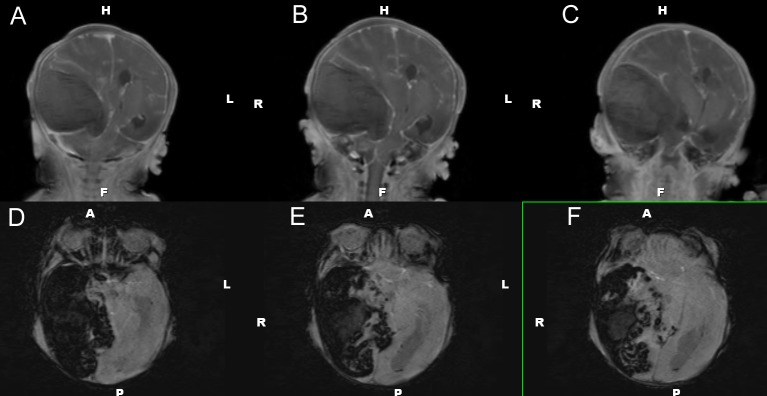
MRI of the brain – T1 coronal views and susceptibility-weighted imaging. T1 coronal (A-C) and susceptibility-weighted imaging (D-F) views characterizing the subdural hematoma.
